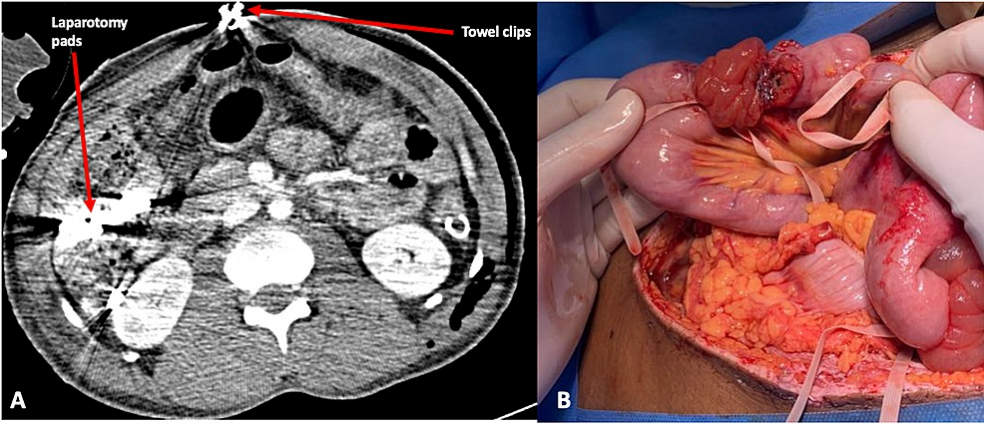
CT findings and intraoperative picture from second-look laparotomy (A) Artefacts seen on CT from towel clips and laparotomy pads; (B) intact bowel ligatures at time of the second laparotomy
